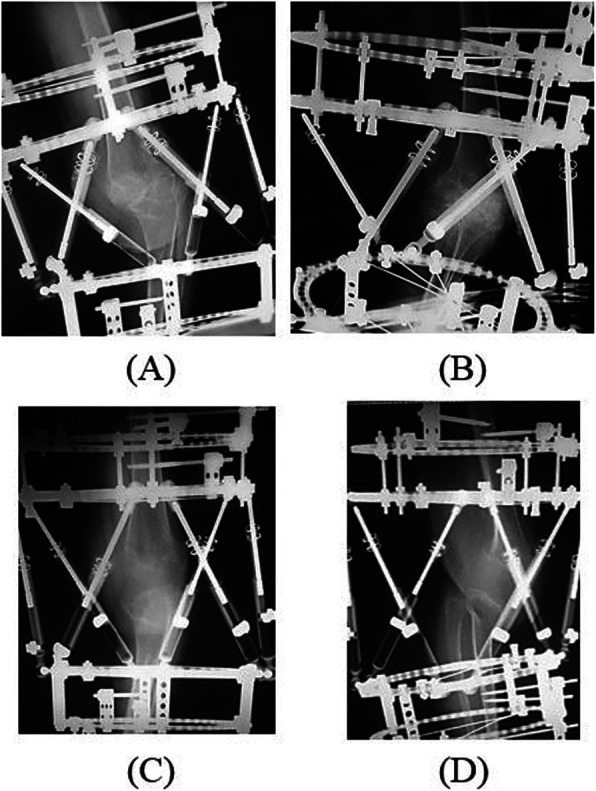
X-ray with circular hexapod external fixation. a Immediate postoperative antero-posterior X-ray with circular hexapod external fixation. b Immediate postoperative medio-lateral X-ray with circular hexapod external fixation. c Antero-posterior X-ray after correction deformity with circular hexapod external fixation. d Medio-lateral X-ray after correction deformity with circular hexapod external fixation
